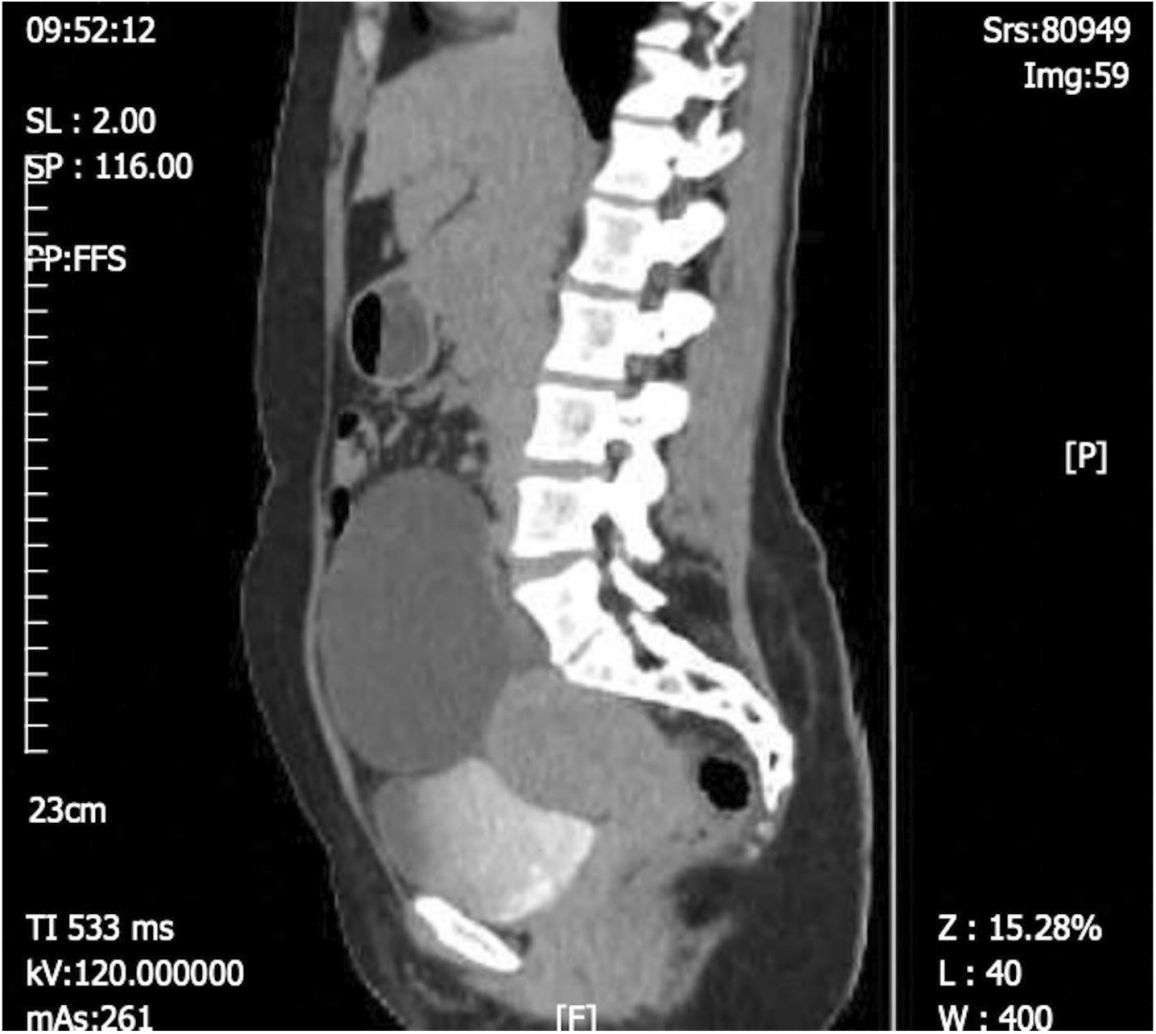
Abdominopelvic computed tomography (CT), sagittal, with intravenous and oral contrast, revealed a large cystic mass arising from the left adnexa with several thin enhancing septa highly suggestive of ovarian cystadenocarcinoma.
